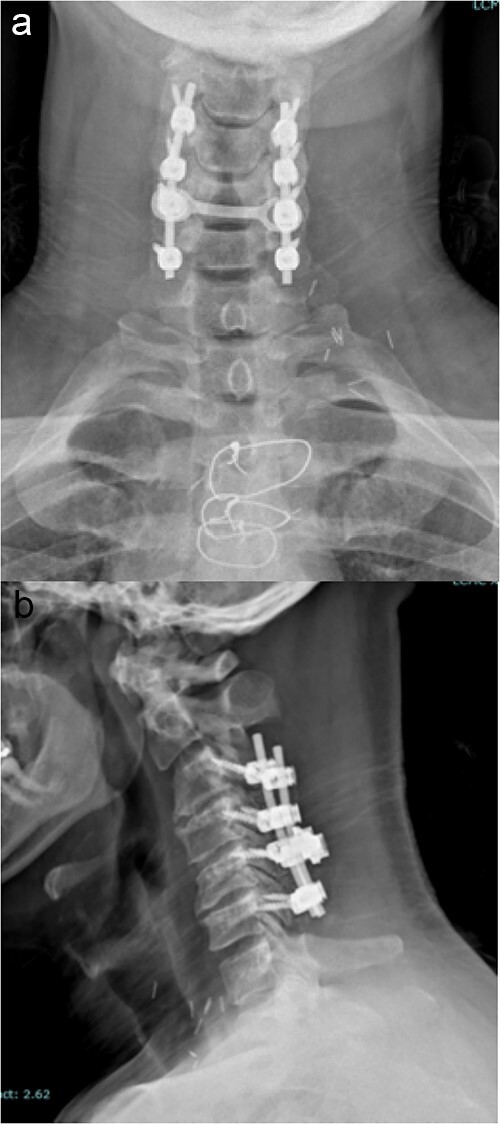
Imaging showing the instrumentation used in a standard PCF. (a) AP X-rays of the cervical spine showing the lateral mass screws and rods inserted during a standard PCF. (b) Lateral X-rays of the cervical spine showing the lateral mass screws and rods inserted during a standard PCF.
